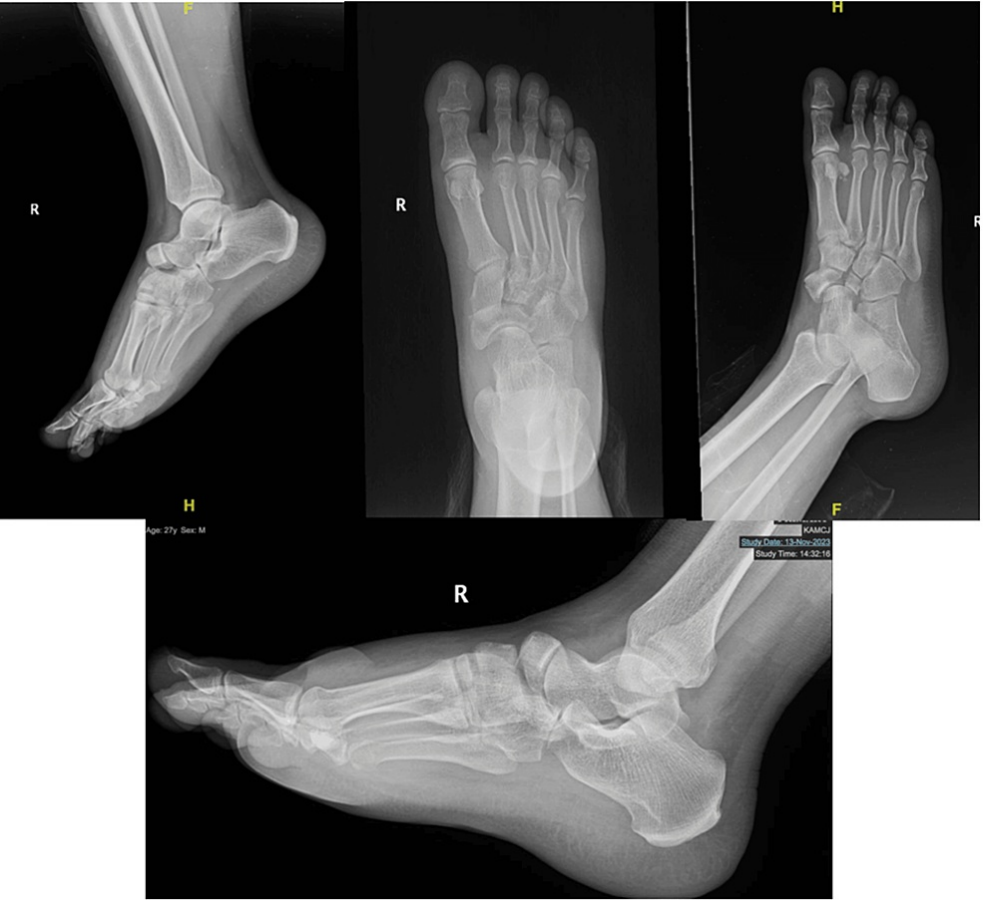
Preoperative X-ray films.
